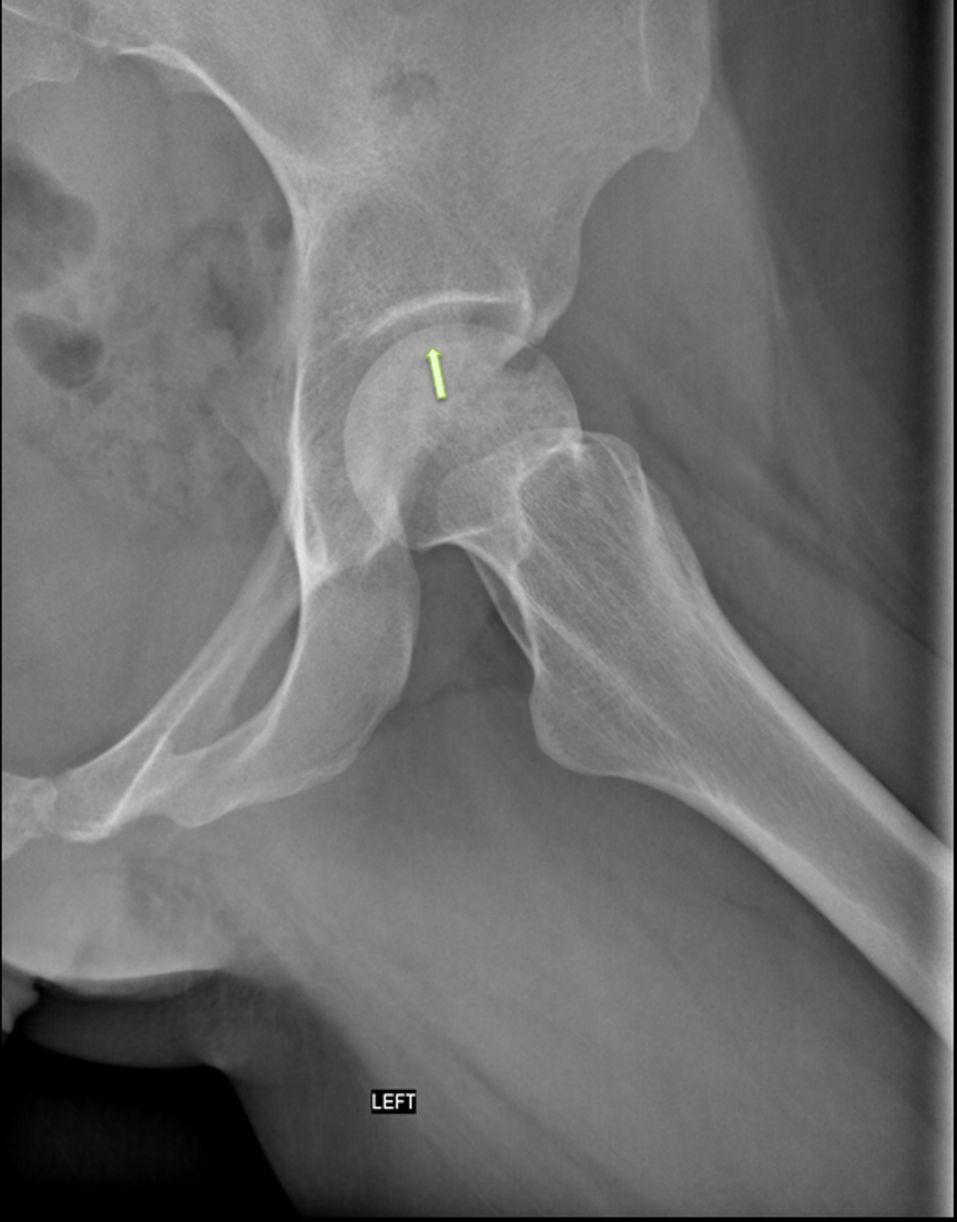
Lateral radiograph of the left hip showing a crescent sign
